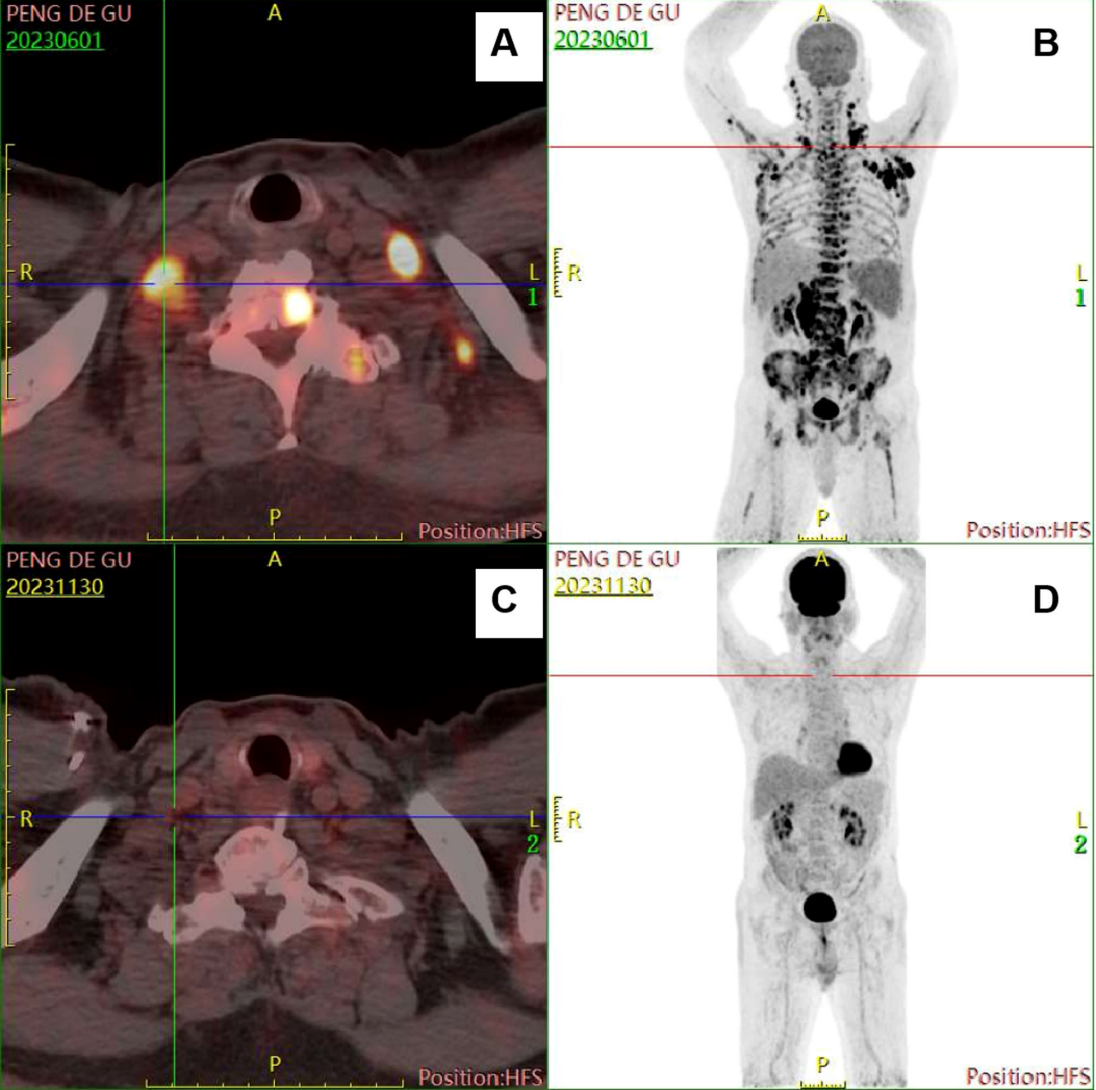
Comparison of images from two PET/CT examinations. (A, B) images when diagnosed with HL-type RS. (C, D) images at the end of chemotherapy treatment.
